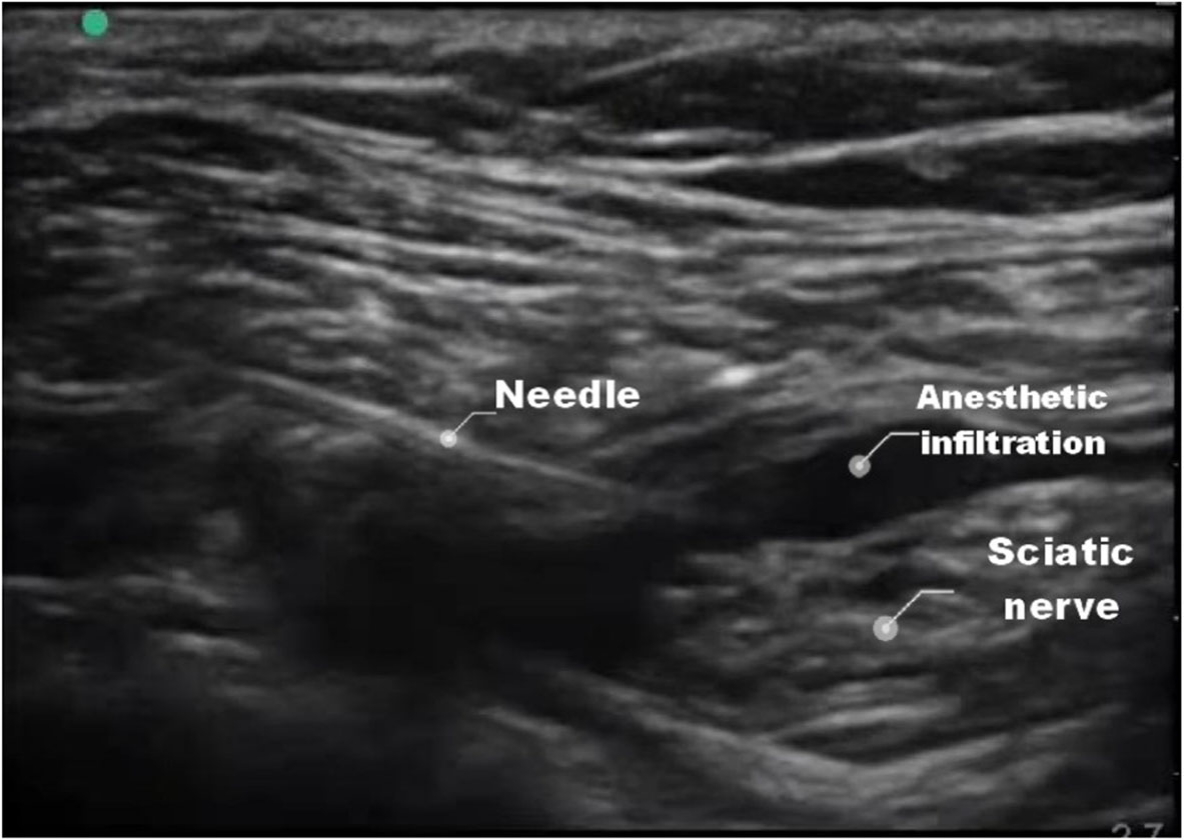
The landmarks of sciatic nerve block and local anesthetic injection under ultrasound view
